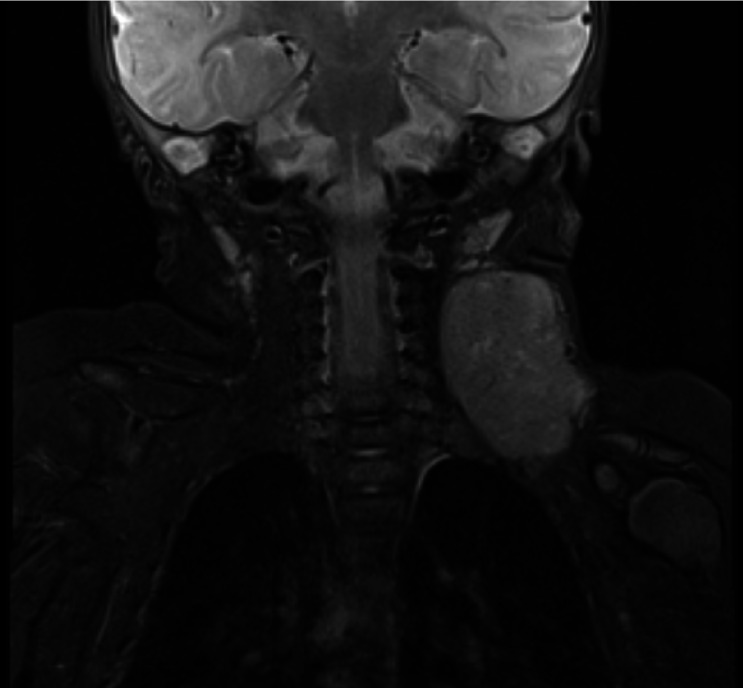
MRI soft tissue neck/thorax coronal plane.
